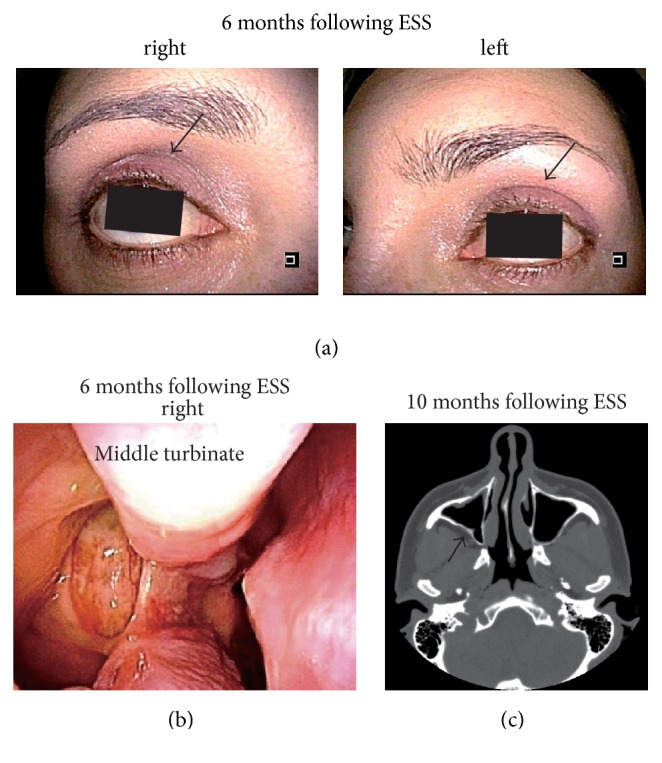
Summary of the clinical examination after the endoscopic sinus surgery. (a) Facial appearance 6 months after the endoscopic sinus surgery (ESS). The arrows in the right and left pictures show the superior sulcus. The deepening of the right superior sulcus that was observed before the sinus surgery appeared to have been eliminated by the ESS. (b) Endoscopic findings 6 months after the ESS. The right uncinate process was removed and recurrence of the deformity was not observed. (c) Computed tomography findings 10 months after the ESS. The deviation of the posterior wall in the right maxillary sinus (shown by the arrow) seems to have been eliminated by the ESS.
